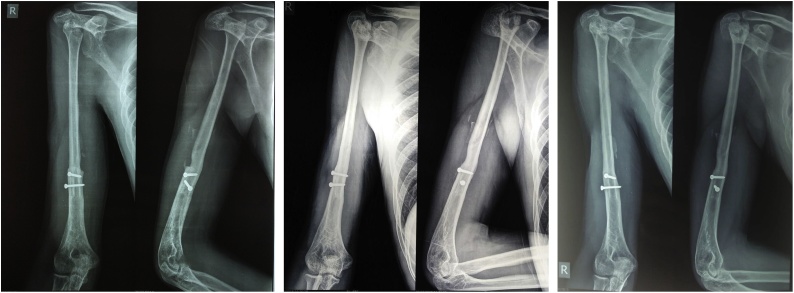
Postoperative plain radiograph: a). 8 months postoperative, b). 2 year post operative, and c).3 years postoperative.
